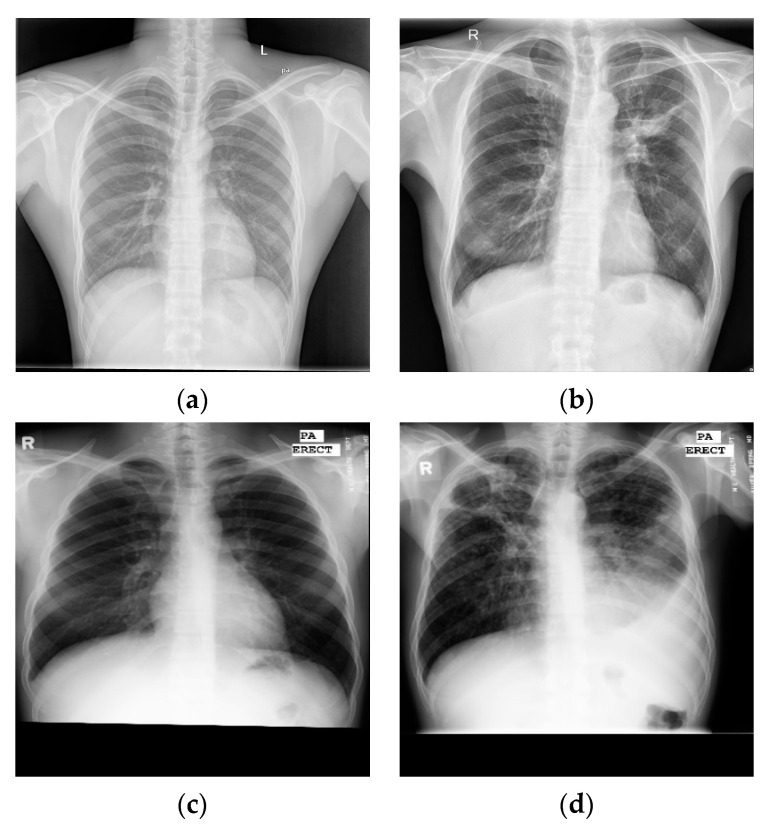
Examples of chest X-ray images from CHN datasets: (a) normal chest X-ray; (b) tuberculosis chest X-ray. Examples of chest X-ray images from MC datasets: (c) normal chest X-ray; (d) tuberculosis chest X-ray.
